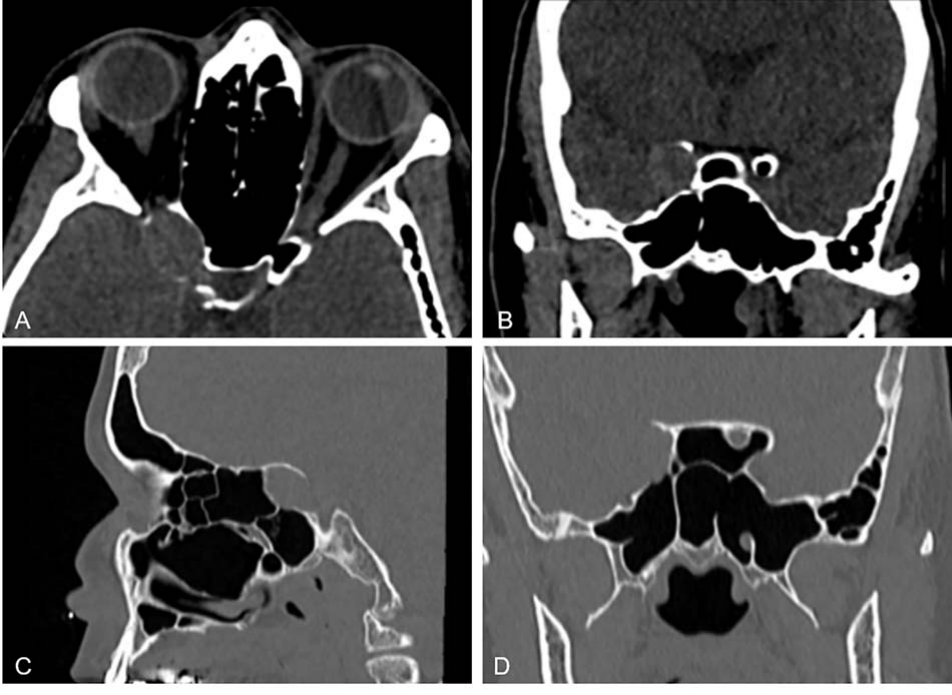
Paranasal sinus CT scans multiplanar reconstructions in axial (A), coronal (B, D) and sagittal (C) planes, with modified window (A and B) and bone algorithm (C and D); they show the right ACP with thin eroded walls, occupied by an oblong, soft tissue density mass that expands the bone cortical and compresses the right orbital apex.
